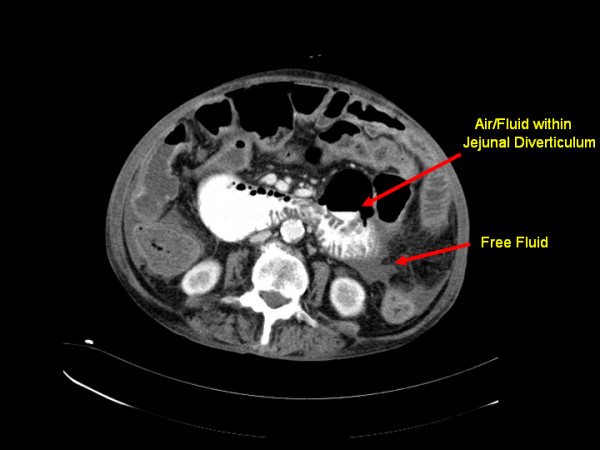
CT scan of abdomen showing thickening of the duodenum and dilatation of the proximal jejunum. Multiple small bowel diverticula were identified with surrounding pockets of free air and fluid adjacent to the jejunal diverticula suggestive of a small bowel perforation.
